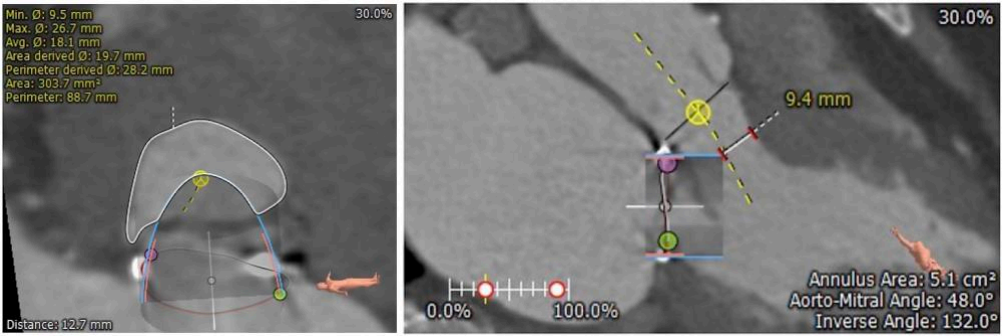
Pre-procedural computed tomography evaluation of the risk of LVOT obstruction. Predicted Neo-LVOT area and aorto-mitral angle based on virtual deployment of a 26 mm Edwards Sapien S3 valve are shown. A neo-LVOT area of 303.7 mm^2^ (>200 mm^2^) and an aorto-mitral angle of 132° (>90°) indicate a low risk of LVOT obstruction.
